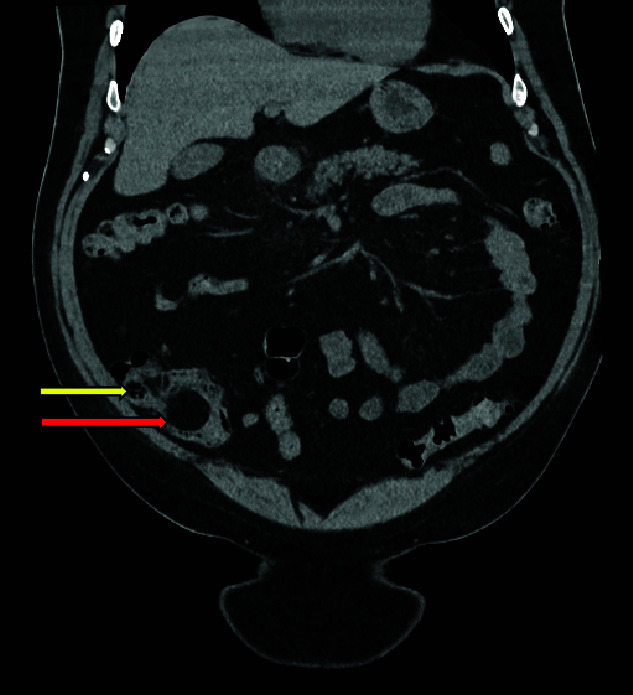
Coronal CT image, showing caecal lipoma (superior arrow) and ileo-caecal valve (inferior arrow).
